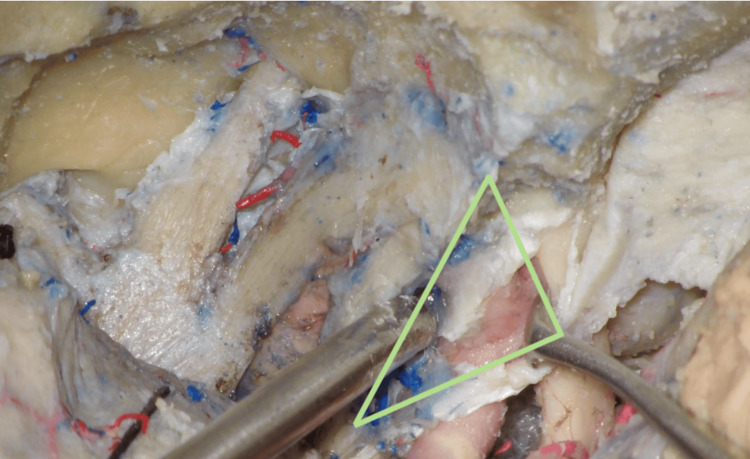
The clinoidal triangle (CT) The triangle limits are the optic nerve medially, the distal dural ring posteriorly, and cranial nerve III laterally. The optic nerve was slightly retracted medially, and cranial nerve III was retracted laterally to expose the internal carotid artery within the triangle.
